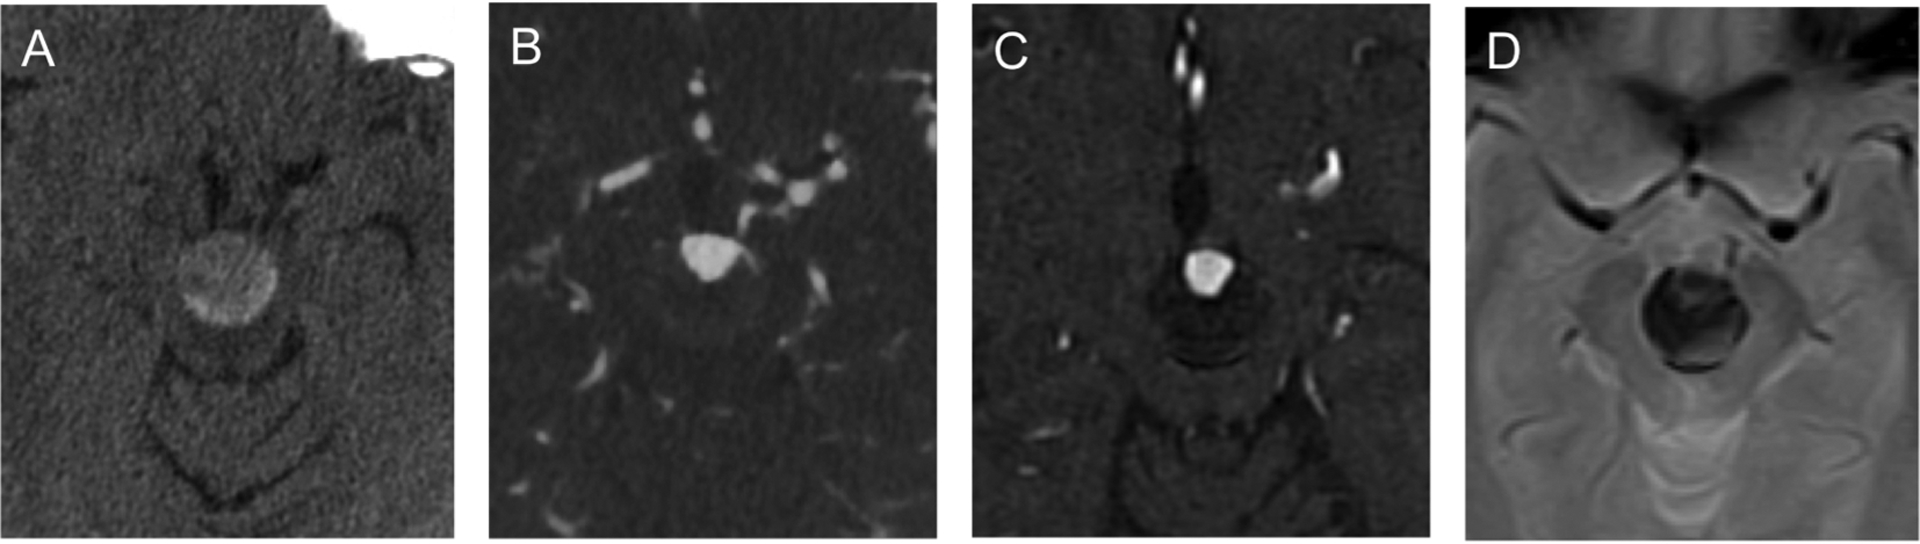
(A) Axial unenhanced CT demonstrates basilar apex aneurysm measuring 20
